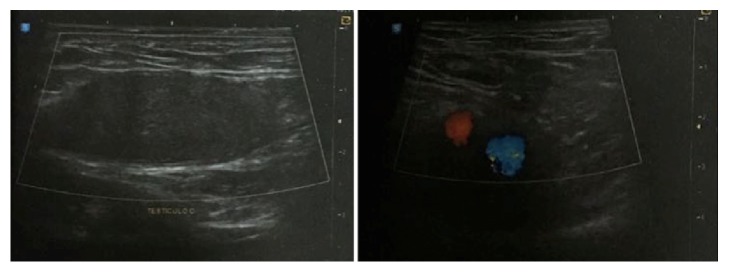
Doppler ultrasound of the right testicle.
